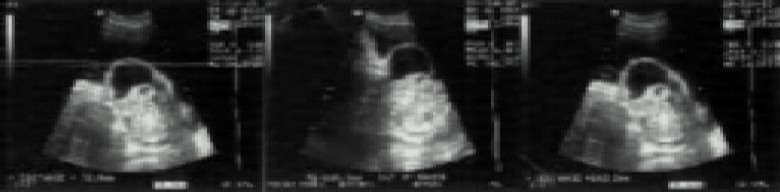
Intrauterine appearance of fetus in ultrasonographic imaging
